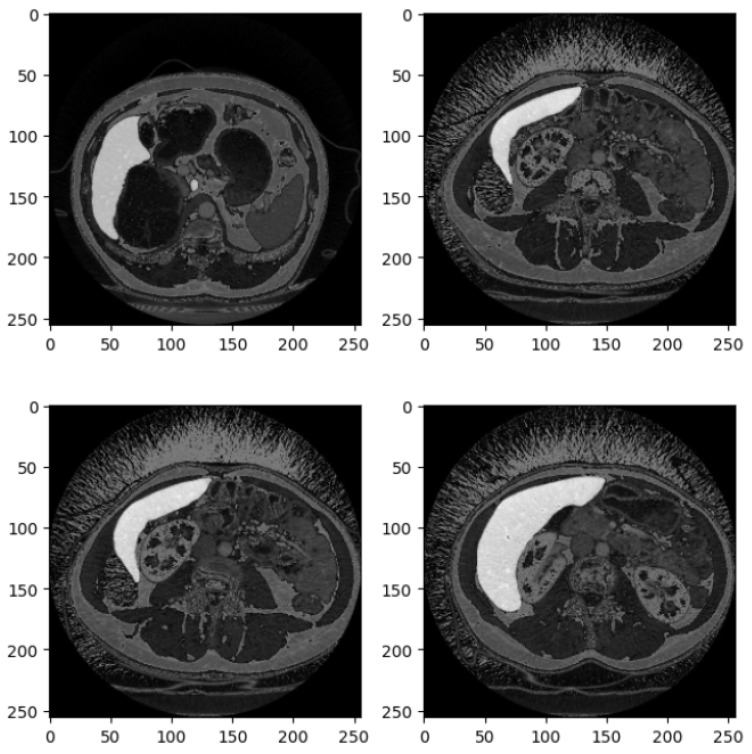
Four examples of CT images with the related mask obtained from the DI dataset.
